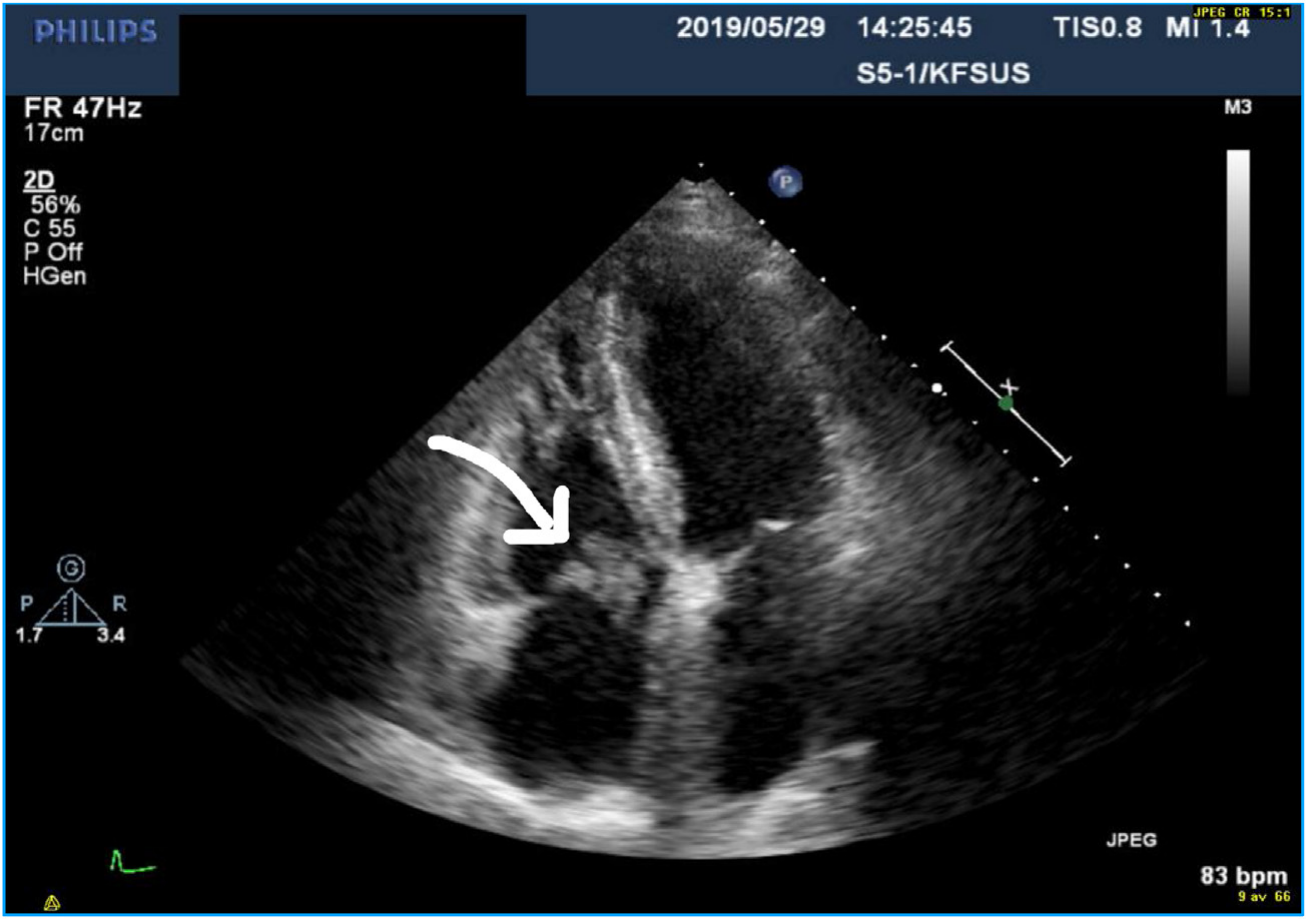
Transthoracic echocardiography demonstrating tricuspid vegetation (arrow).
